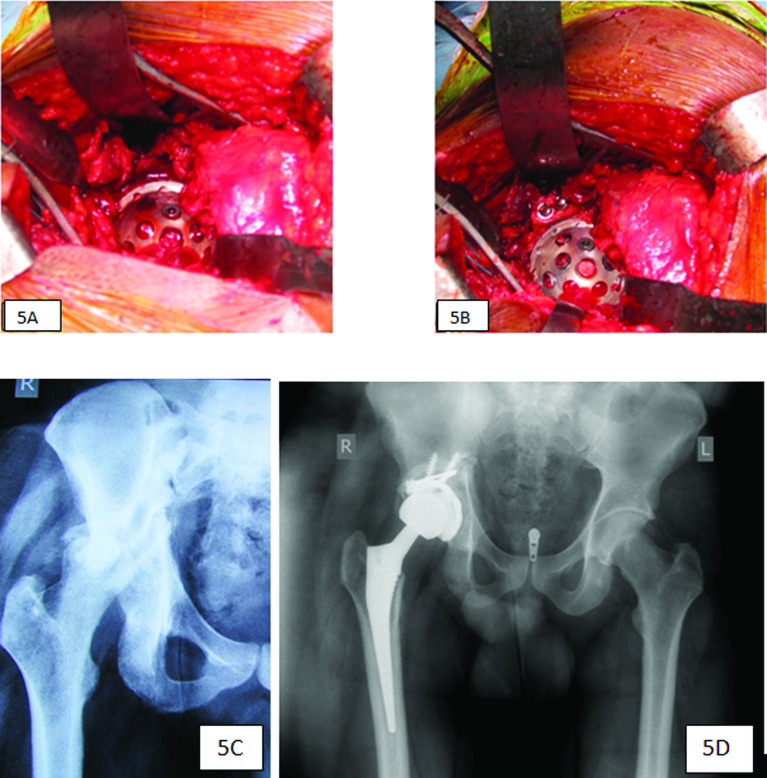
(A) Superior wall defect. (B) Superior defect reconstruction with a structural bone graft and screw fixation. (C) Radiograph showing a superior wall fracture with femoral head subluxation. (D) Radiograph showing good bone graft incorporation at five years.
